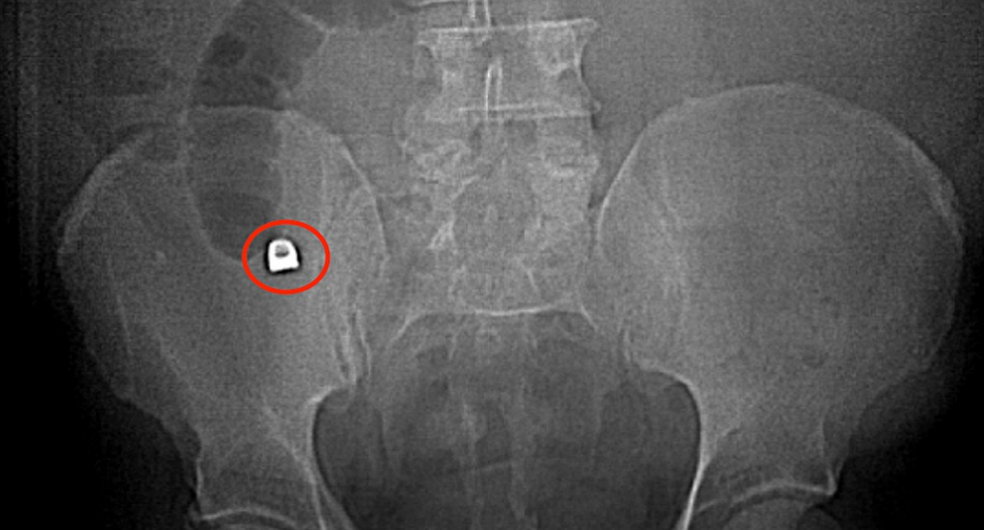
Plain film showing metallic object in appendix
